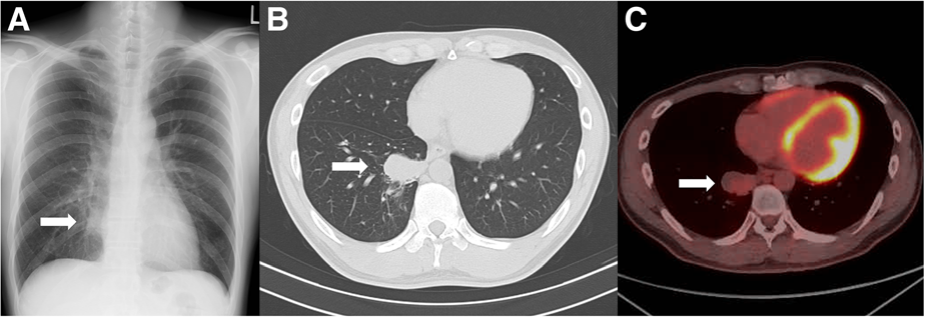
Images of the lung mass. a Chest X-ray on the first visit to our hospital. A mass lesion was shown in the right lower field (arrow), b Chest computed tomography on the first visit to our hospital. A single mass lesion (4 cm in size) was shown in the right lower lobe of the lung (arrow), c Fluorodeoxyglucose-positron emission tomography (FDG-PET) before the surgery, 4 months after the first visit. A single mass lesion was still remained in spite of the antibiotic treatment. It had abnormal uptake with a maximal standardized uptake value (SUV max) of 2.51 (arrow)
